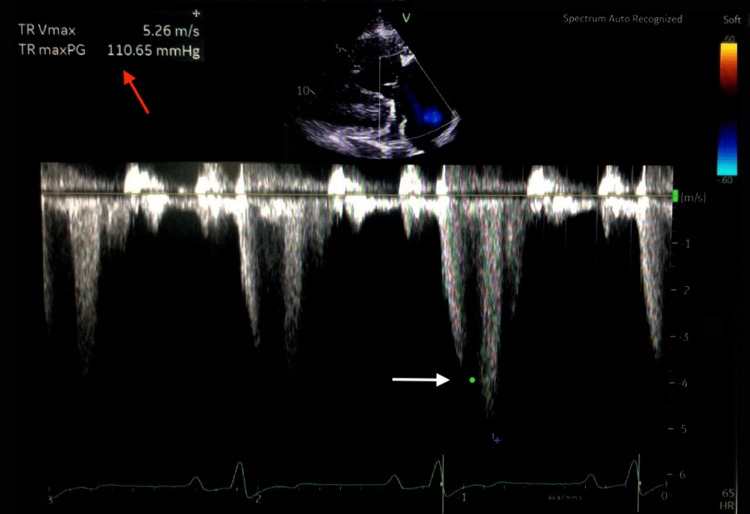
Trans-thoracic echocardiography with continuous-wave Doppler at the tricuspid valve Shows markedly elevated regurgitant jet velocity (white arrow). The calculated TRVmax was 5.26 m/s with a pressure gradient of 110 mmHg (red arrow), findings consistent with severe pulmonary hypertension. PG: pressure gradient; TR: tricuspid regurgitation; TRVmax: tricuspid regurgitation maximal velocity.
